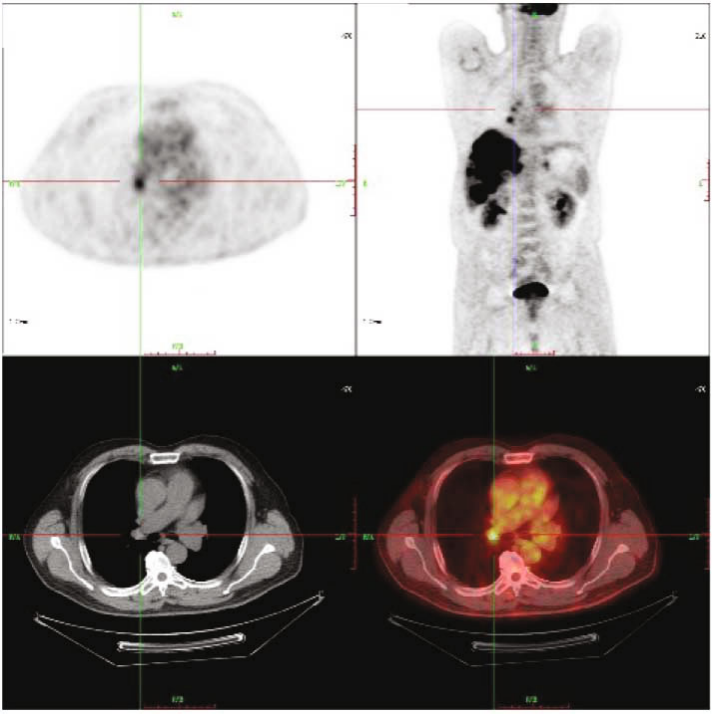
CT, PET, and PET/CT original images.
